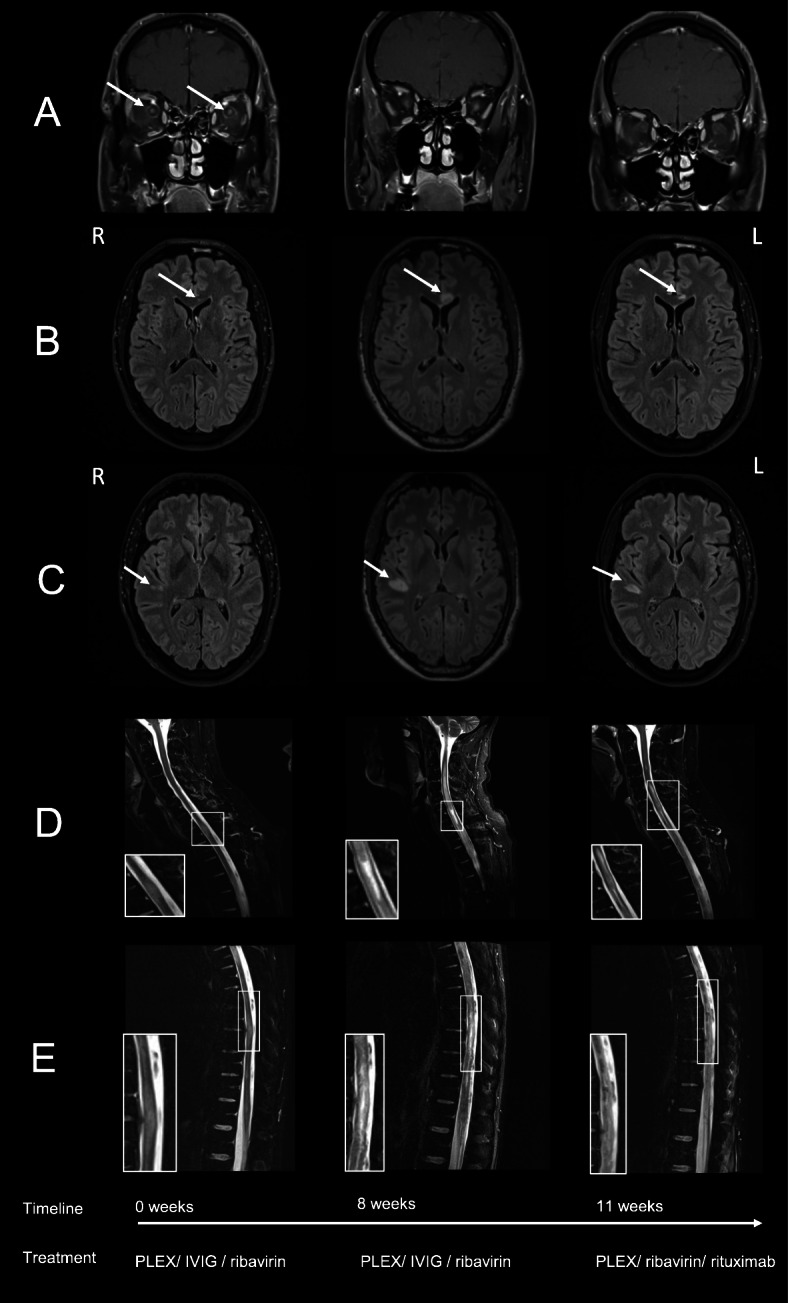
Radiological findings in the course. Brain MRI of hepatits-E-related lesions displayed in a time chart over 11 weeks (from left to right). a Retrobulbar enhancement of nervi optici which shows a regredient state, coronal T1-weighted images with contrast medium. b, c Hypersignal of the brain marrow of the temporal lobe and rostral of the corpus callosum on the left which shows a deterioration in the second picture. After 11 weeks a decline of the lesions were found (FLAIR). d A 10 mm hyperintense lesion at the cervical spine was found and declared as a severe edema which caused swelling. The middle picture shows a significant deterioration of the lesion. The right picture shows a partial decline of the lesion (T2, turbo inversion recovery magnitude (TIRM)-weighted images without contrast medium). e Significant space-consuming hyperintense lesion of the thoracic spine was detected in the first MRI (left picture). Middle picture shows a distinct progredient edema. The right picture shows a significant regression of the pathologies. (TIRM-weighted images without contrast medium). Investigations were performed with 3.0 Tesla, Siemens Magnetom Verio for the left and right pictures and for the middle pictures: 3.0 T Siemens Magnetom Vida was used
